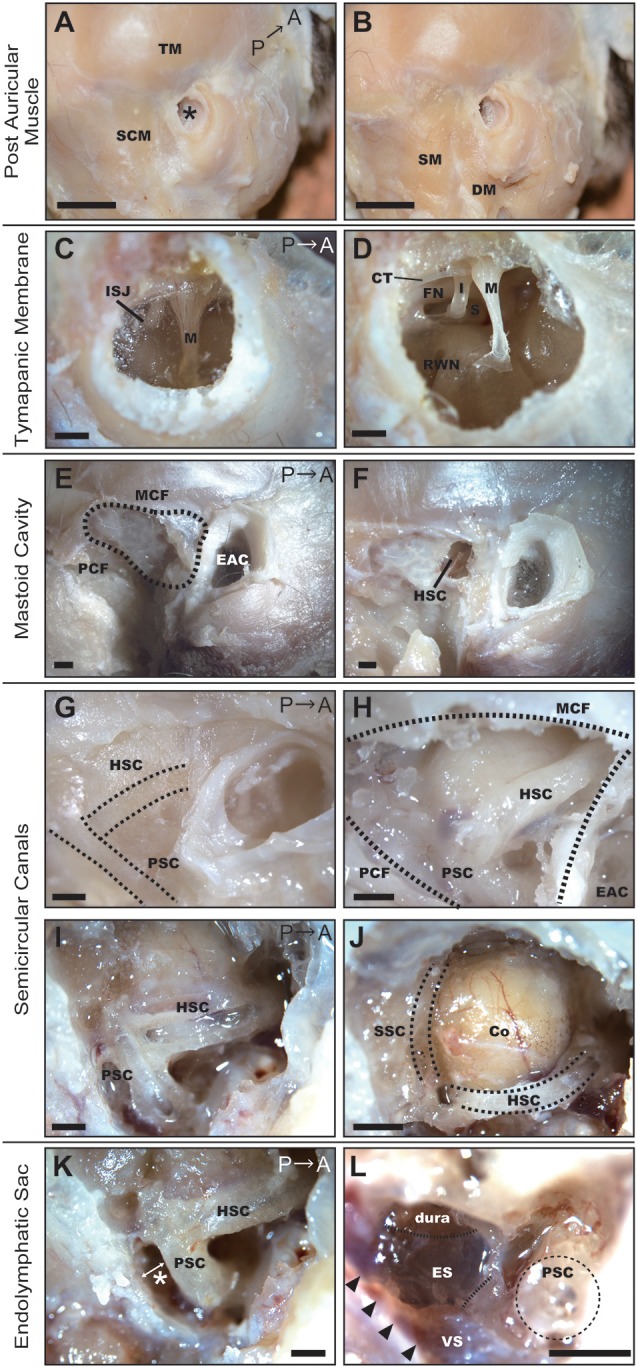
Dissection of the right temporal region of the common marmoset. (A) Postauricular muscles detected after the removal of skin and subcutaneous tissue. SCM, sternocleidomastoid muscle; TM, temporal muscle; Asterisk, EAC. Scale bar, 10 mm. (B) Muscles after the removal of the SCM. SM, splenius capitis muscle; DM, posterior belly of the digastric muscle. Scale bar, 10 mm. (C) Tympanic membrane as viewed from the EAC. M, malleus; ISJ, joint of incus and stapes. Scale bar, 1 mm. (D) The view from the EAC after extracting the tympanic membrane. M, malleus; I, incus; S, stapes; FN, facial nerve; CT, chorda tympani nerve; RWN, round window niche. Scale bar, 1 mm. (E) Temporal bone after removal of postauricular muscles. MCF, middle cranial fossa; PCF, posterior cranial fossa; EAC, external auditory canal. Scale bar, 1 mm. (F) Hole drilled on the temporal bone surface. HSC, horizontal semicircular canal. Scale bar, 1 mm. (G) The temporal bone of a neonatal common marmoset. Dotted lines indicate the HSC and posterior semicircular canal (PSC) as observed through the temporal bone. Scale bar, 1 mm. (H) Semicircular canals of a 6-month-old common marmoset. Scale bar, 1 mm. (I) Semicircular canals after canalostomy. Scale bar, 1 mm. (J) The cerebellar cortex (Co) protruding inside the HSC and superior semicircular canal (SSC). Scale bar, 1 mm. (K) The entry to manipulate the endolymphatic sac (ES). It was necessary to open the space between the PSC and the posterior wall of the mastoid cavity (asterisk). Scale bar, 1 mm. (L) ES after drilling out the PSC and stripping the petrous portion of the temporal bone. Arrowheads indicate the venous sinus (VS). Scale bar, 1 mm.
